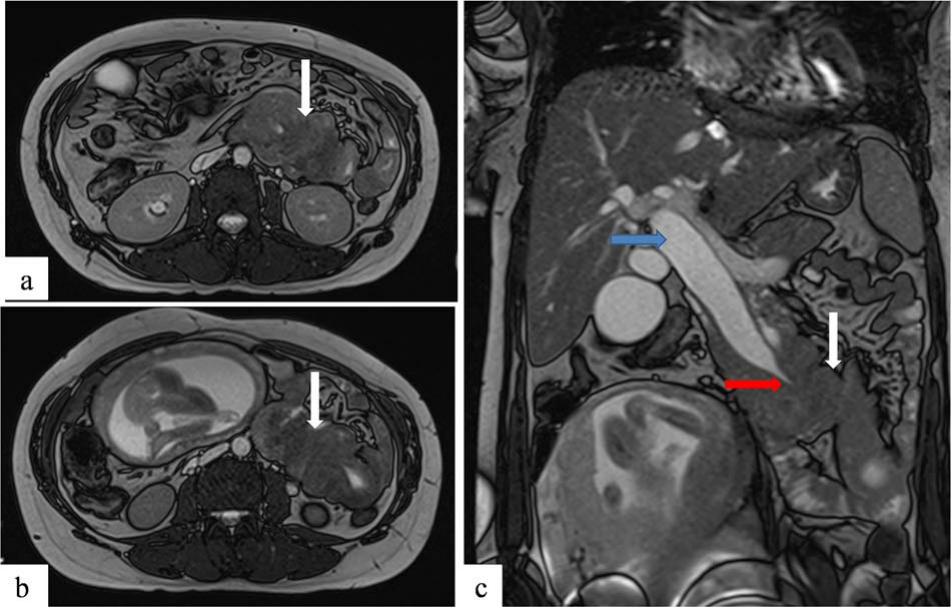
Axial (a, b) and coronal (c) T2-weighted TrueFISP MR images confirming the duodenojejunal intussusception (white arrows) and showing inclusion of the terminal bile duct within the intussuscepted segment (red arrow), resulting in significant upstream biliary dilatation (blue arrow).
